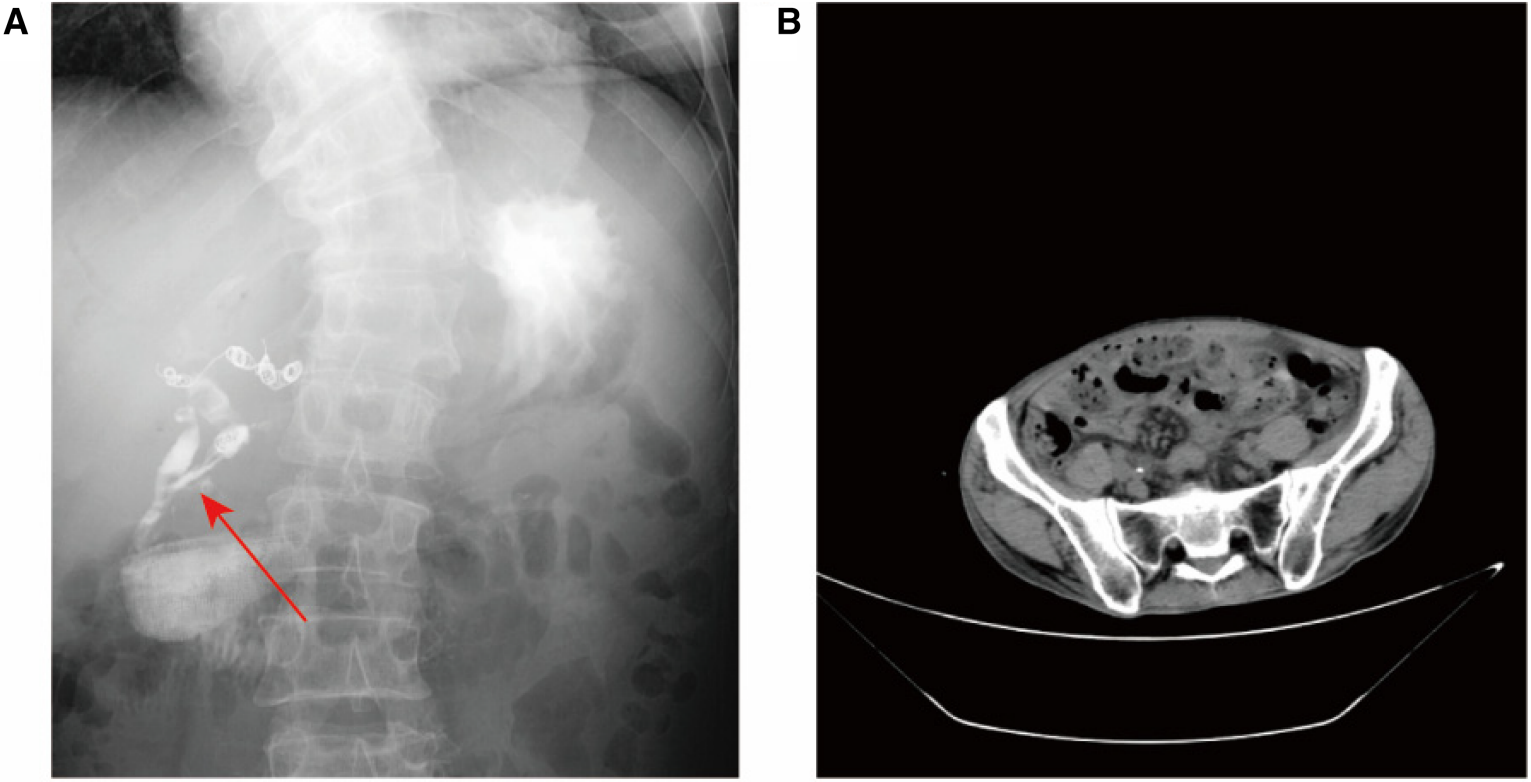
Imaging examination. (A) Coronal radiographic image of the fistula or fistula tract before the patient's treatment was blocked. Fistula tract connected to abdominal wall (shown by arrow), scoliosis; (B) Abdominal CT suggests ureteral calculus.
